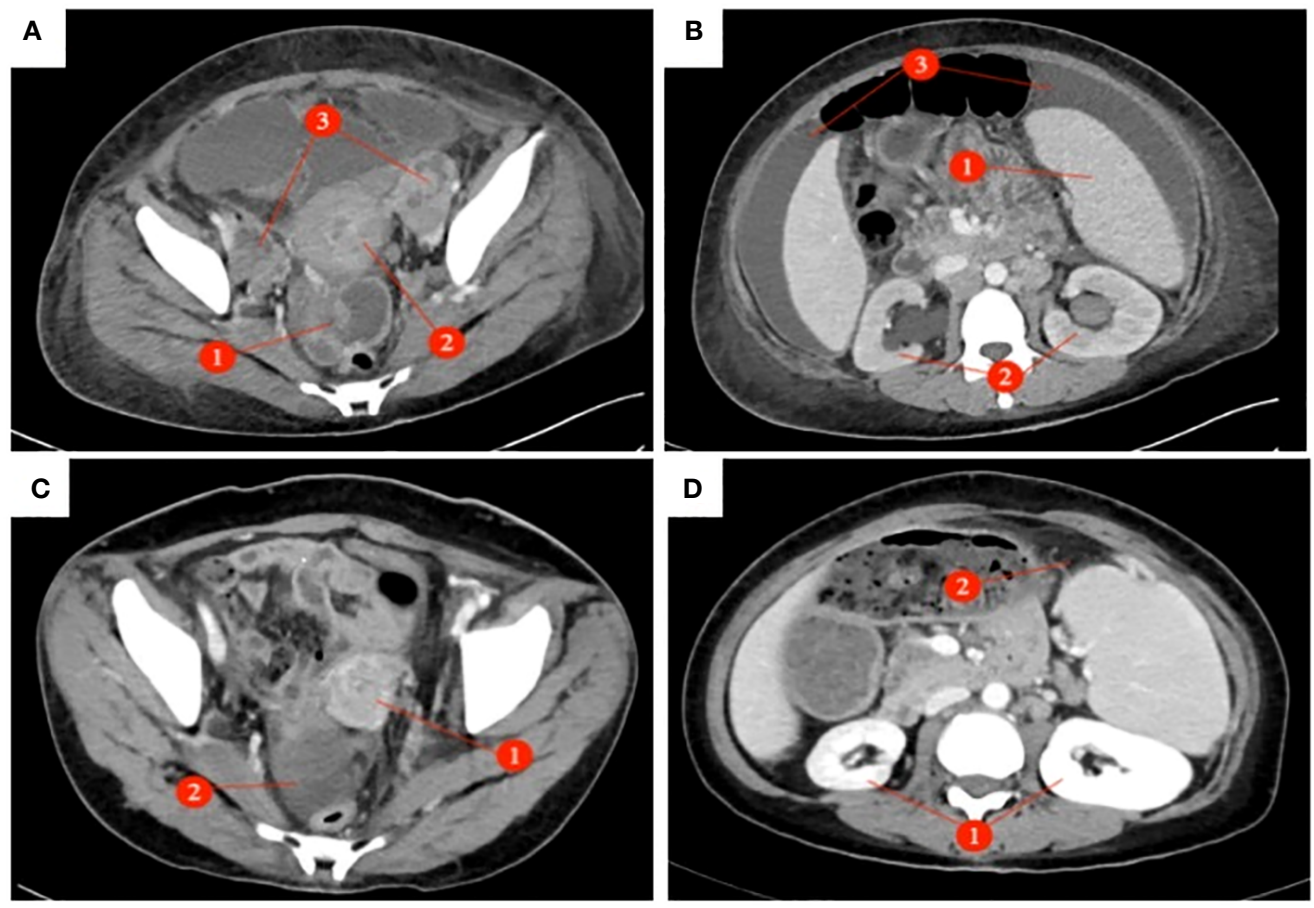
Abdominal and pelvic enhanced CT: (Before chemotherapy AB After chemotherapy CD) : (A) The pelvic mass presents as an irregular solid cystic lesion measuring 5×6cm, exhibiting patchy shadowing and demonstrating enhancement of both the solid component and cyst wall. (①); The cervix exhibited a 5 x 4cm mass with heterogeneous enhancement of soft tissue density. (õ②); Bilateral ovarian enlargement (③), Pelvic effusion. (B) Splenomegaly (①); bilateral hydronephrosis with bilateral hydrourete (②), Abdominal effusion (③). (C) Disappearance of cervical lesion (①), In the right adnexal area, the density shadow of irregular soft tissue mass was 4.1 x 4.3cm (②). (D) Disappearance of bilateral hydronephrosis (①), Splenomegaly, Splenic abscess (5.5×1.7cm) (②).
